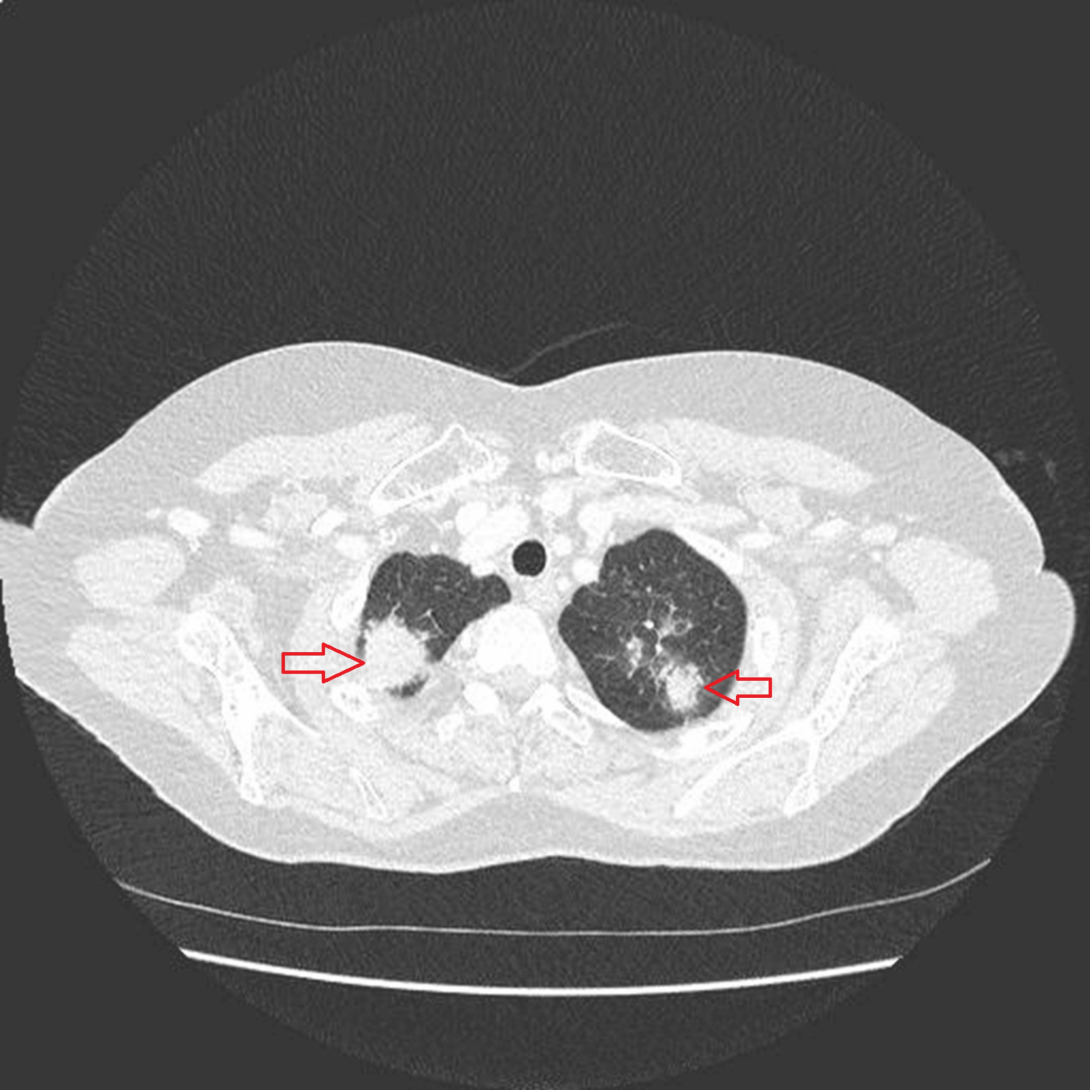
CT scan showing bilateral apical opacification
